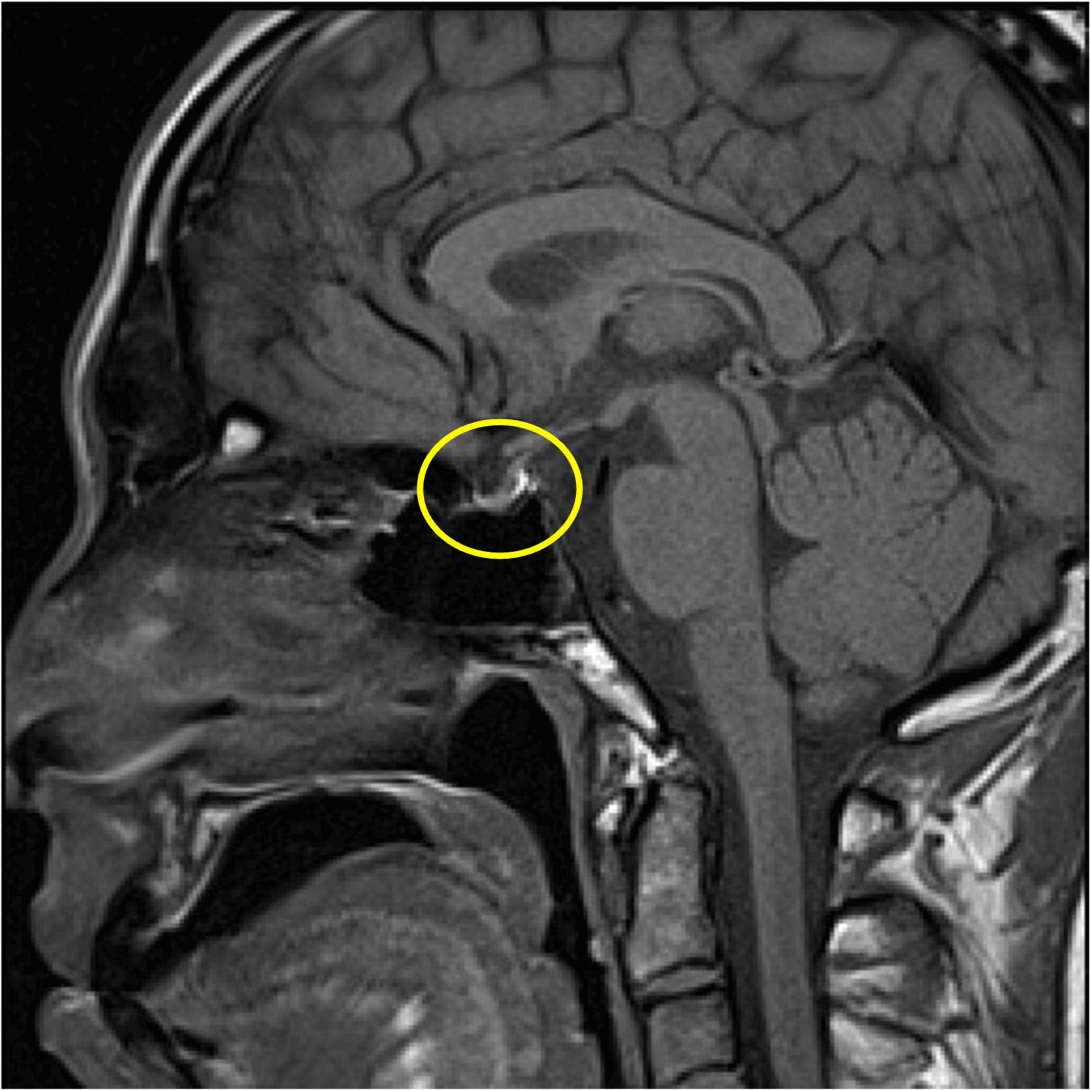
Contrast-enhanced MRI scan of the brain Sagittal T1-weighted MRI of the brain. The pituitary gland appears normal in size and contour. No evidence of swelling or mass lesion is observed.
